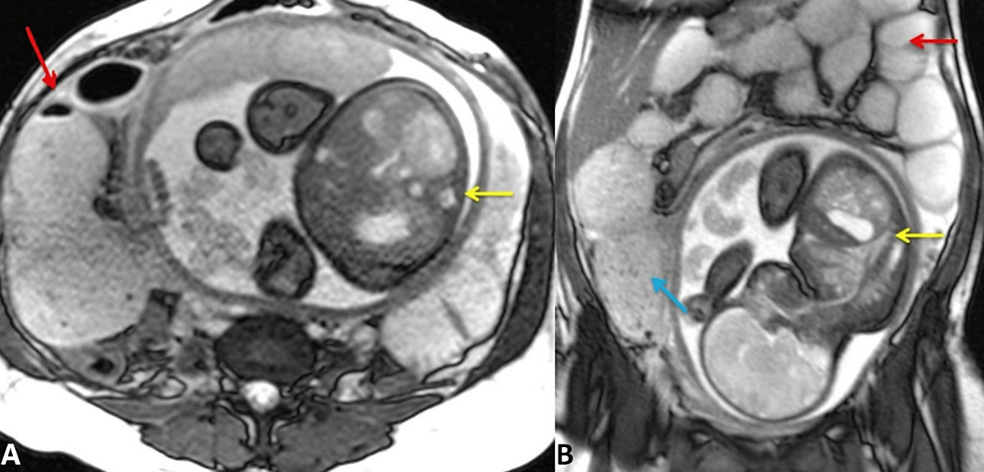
MRI showing (A, axial view) a single intrauterine fetus (yellow arrow) and a dilated segment of the ileum in the right iliac fossa (red arrow) and (B, sagittal view) a dilated segment of the ileum in the right iliac fossa (blue arrow), a single intrauterine fetus (yellow arrow), and a dilated small bowel (red arrow). MRI: magnetic resonance imaging.
